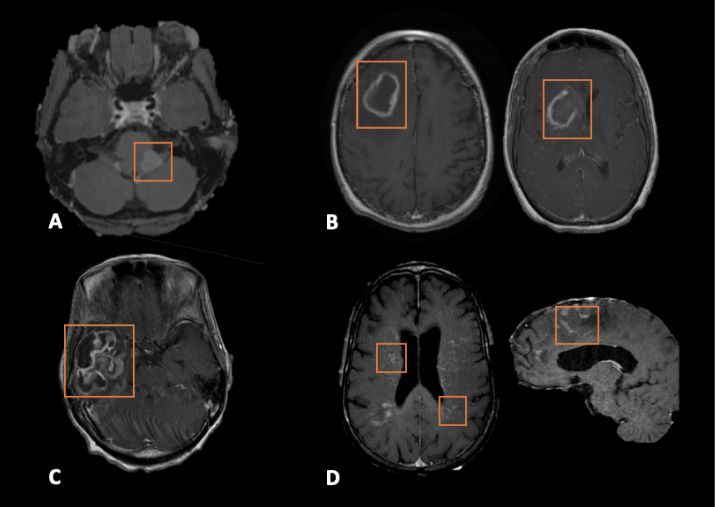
Examples of enhancement types on contrast-enhanced T1-weighted sequences. A Homogeneous enhancement. B Ring enhancement (left: complete ring, right: open ring); C Necrotic, heterogeneous enhancement. D Leptomeningeal enhancement (left: Virchow–Robin enhancement, right: leptomeningeal)
